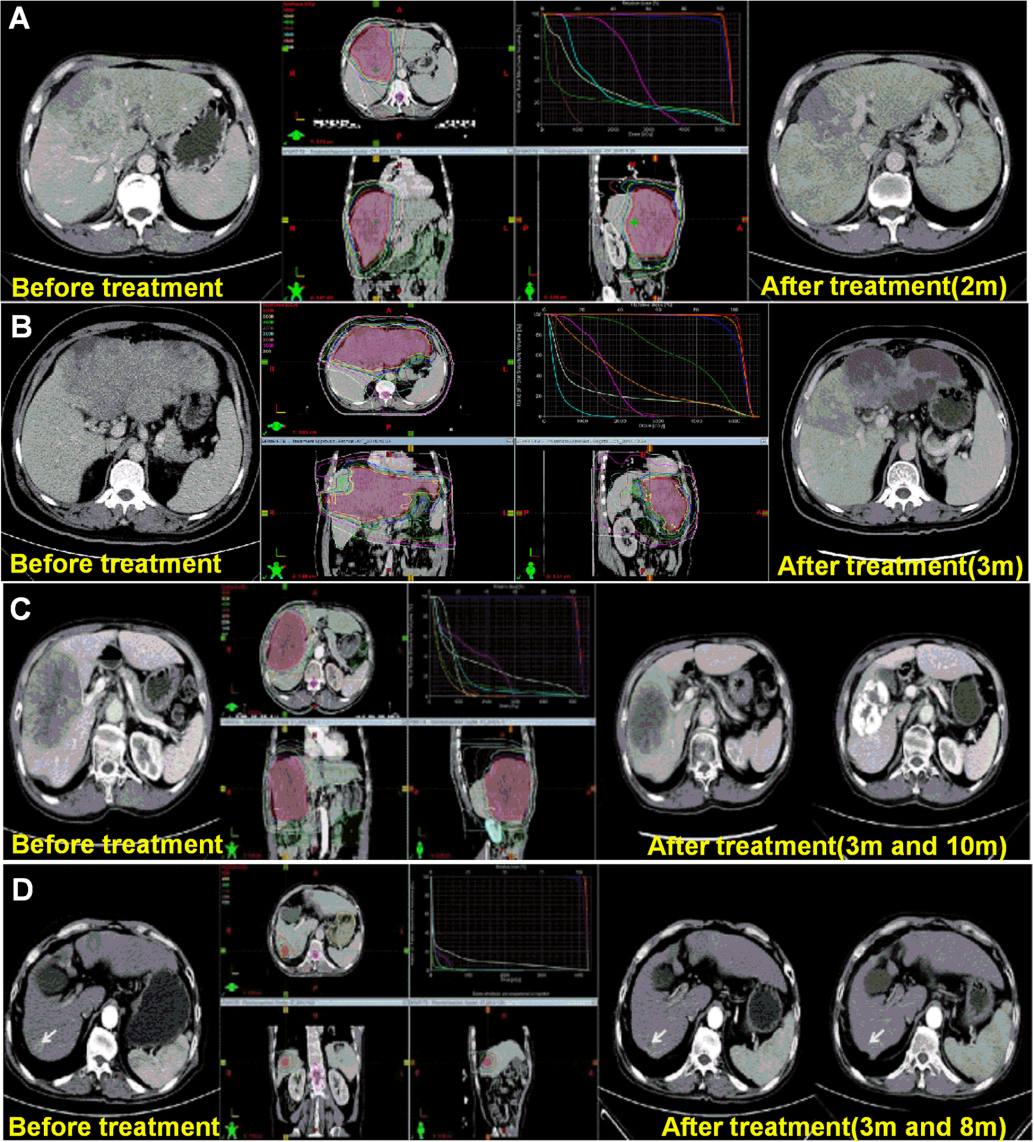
Radiotherapy has a good effect on advanced liver cancer. a-d Intensity-modulated radiotherapy (IMRT) was used to deliver 5000 cGy to the planning target volume (PTV) in 25 fractions, given once a day over 32 days. a CT scans before treatment (*left*) and at 2 months after treatment (*right*). b CT scans before treatment (*left*) and at 3 months after treatment (*right*). c CT scans before treatment (*left*), at 3 months after treatment, and at 10 months after combination transarterial chemoembolization(TACE) treatment (*right*). d CT scans before treatment (*left*) and at 3 months and at 8 months after treatment (*right*)
